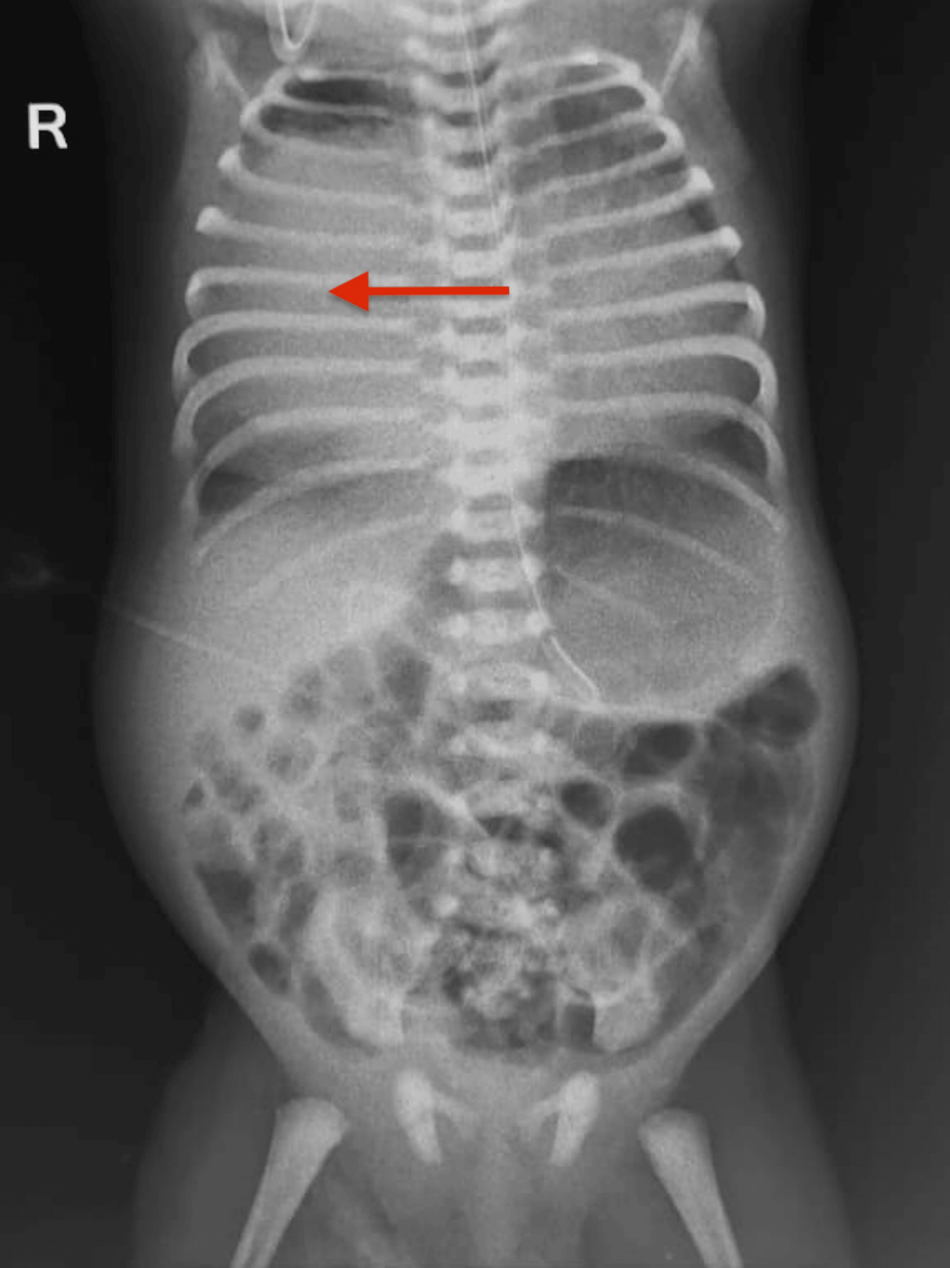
Chest X-ray showing a well-defined, homogeneous opacity (arrow) predominantly involving the middle zone of the right lung, causing a tracheomediastinal shift to the left.
